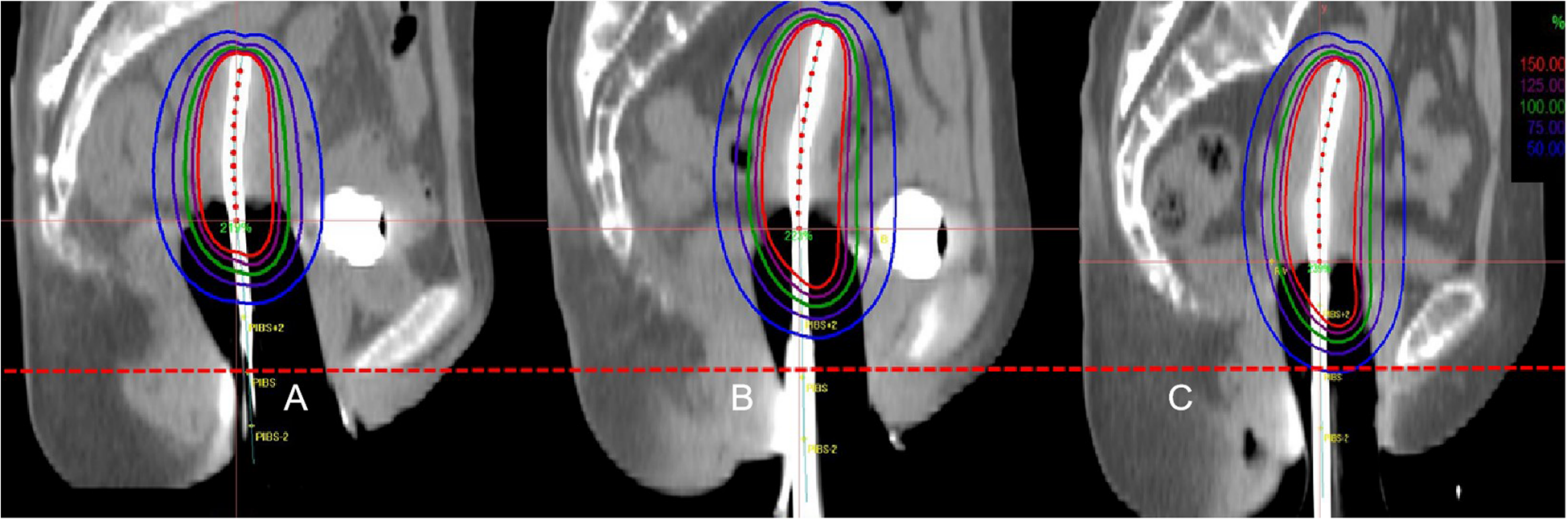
BT CT sagittal view for a patient with tandem and ovoid applicator. The picture shows three cases of BT planning for three patients with different VRLs. a: Patient with a VRL of 6.8 cm. b: Patient with a median VRL of 4.5 cm. c: Patient with a VRL of 3.5 cm, in whom the PIBS+ 2 cm point was located in the high dose area. Red contour: the 150% isodose line. Green contour: the 100% isodose line. Red dashes: level of PIBS points. Yellow dot: PIBS+ 2 cm point. With a shorter VRL, the PIBS+ 2 cm point becomes closer to the vaginal applicator. Definition of the PIBS+ 2 cm is not applicable anymore
